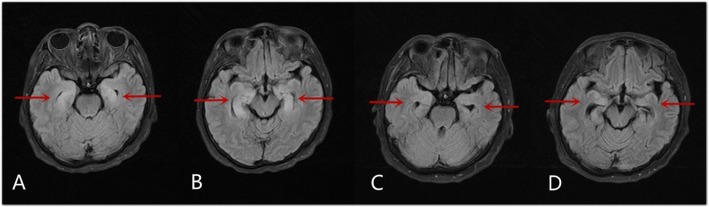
Magnetic resonance imaging of Case two. a-b Magnetic resonance imaging (fluid-attenuated inversion recovery sequence; FLAIR) shows abnormal signals in the bilateral hippocampus, as indicated by the red arrows. c-d Repeated magnetic resonance imaging 7 months later shows significantly improvement
